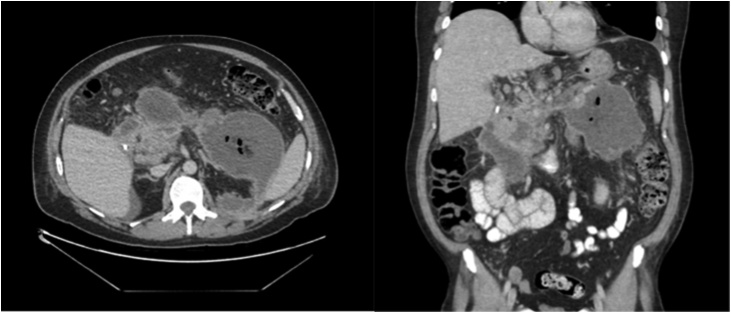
CT scan of the abdomen before drainage. This scan shows a pancreatitis and necrosis with air configurations in peripancreatic fluid collections.
